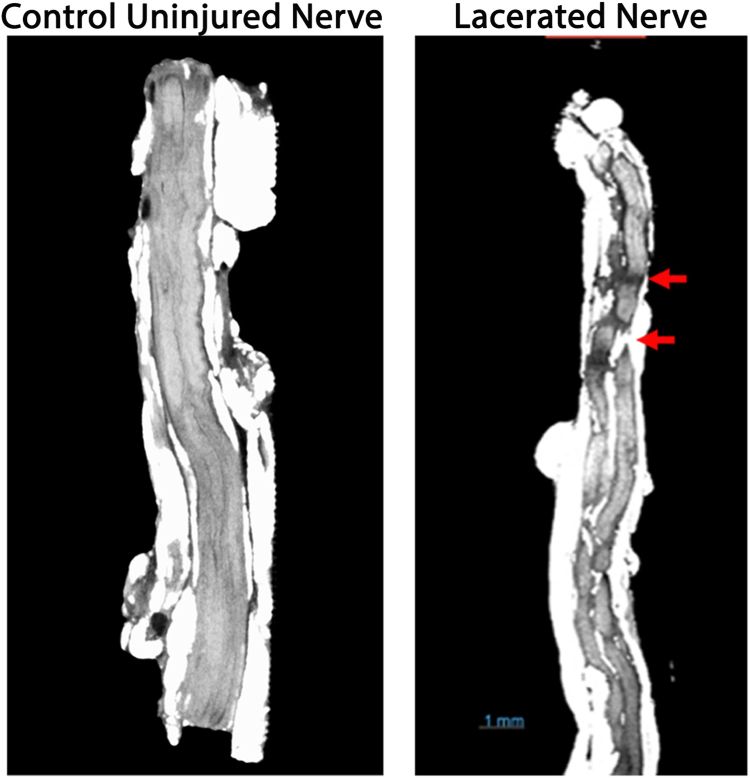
Representative radiographs. Control uninjured nerve samples were used as the benchmark for nerve imaging of lacerated nerves. Red arrows indicate areas of “skip” lesions, indicating damage.
